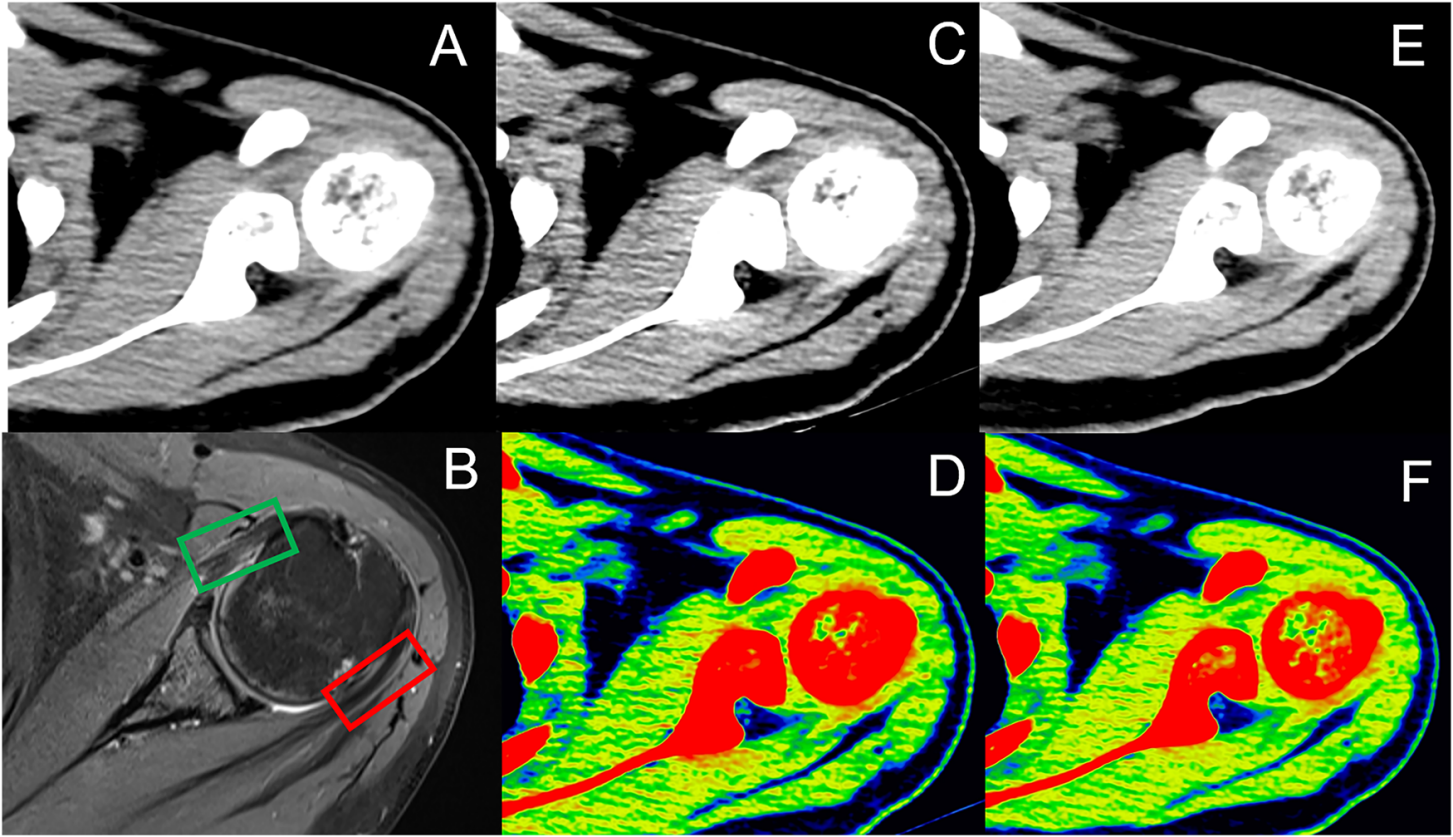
Transverse plane images of a 46-year-old woman with normal left infraspinatus tendon and degenerative subscapularis tendon: (A) SCT image, (B) proton density weighted fat-saturated MRI image displaying a normal infraspinatus tendon (red rectangular box) and a degenerated subscapularis tendon (green rectangular box), (C) DECT images with mono+ 50 keV, (D) color-coded DECT images with mono+ 50 keV, (E) DECT images with mono+ 90 keV, and (F) color-coded DECT images with mono+ mode90 keV. SCT, standard computed tomography; DECT, dual-energy computed tomography; MRI, magnetic resonance imaging
